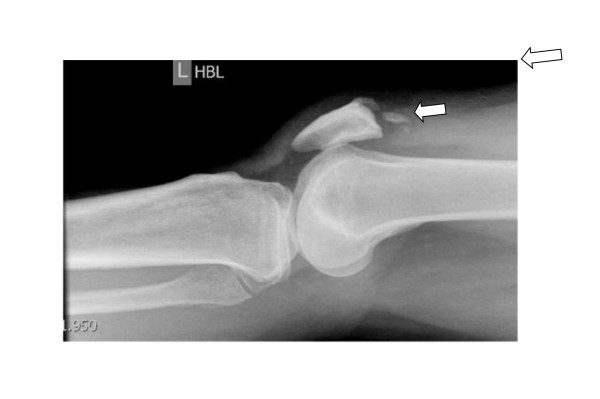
Radiograph of the left knee showing quadriceps rupture.
